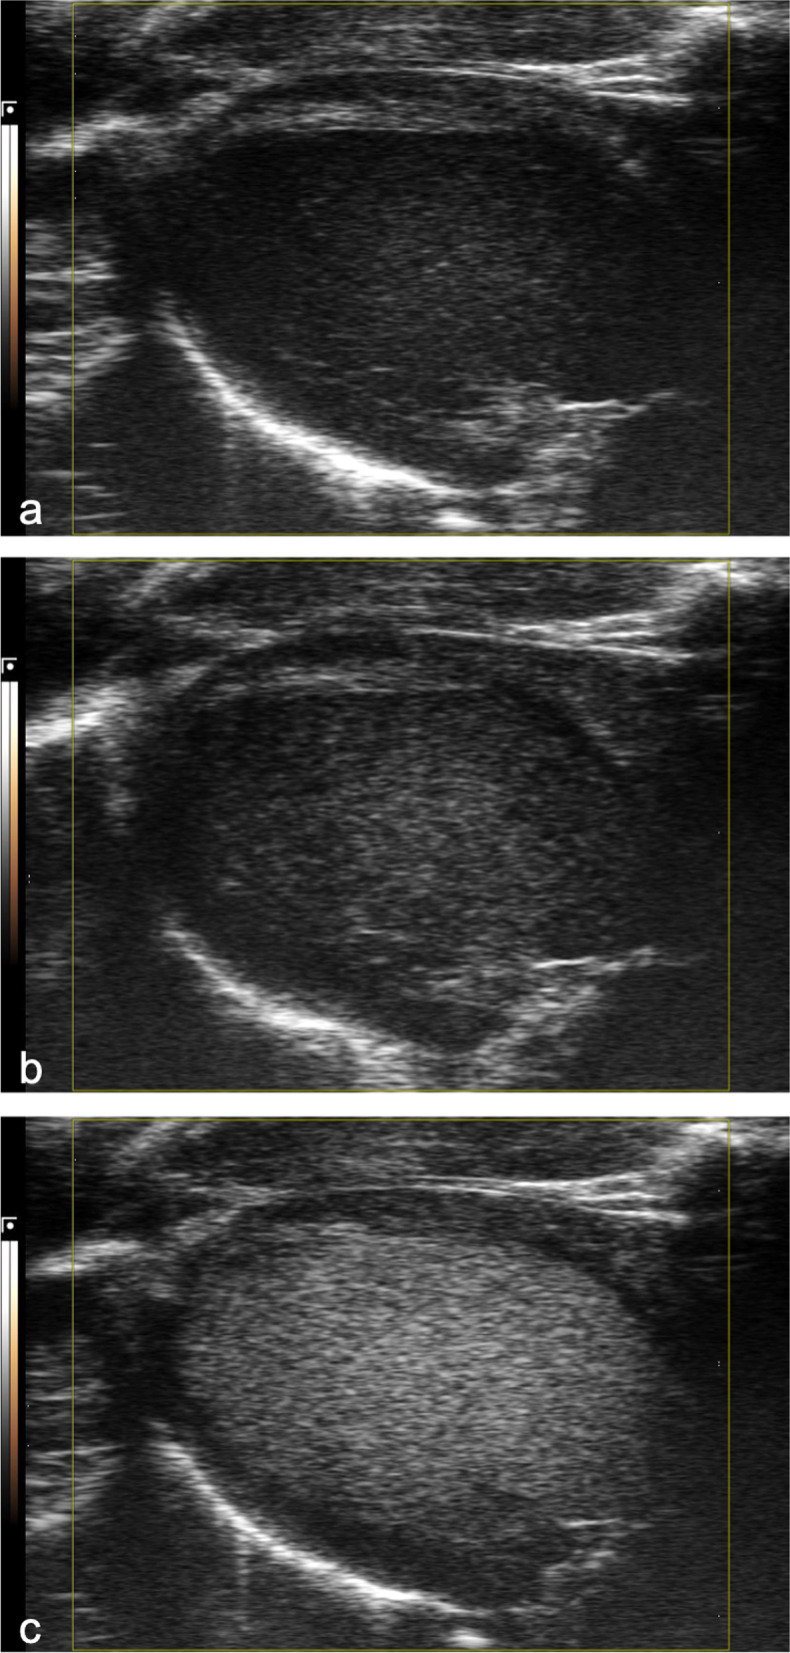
Representative time course of contrast activity in the lumen of the left ventricle. Shown are systolic gated modified parasternal long axis images that were acquired during nonlinear contrast imaging at the time of contrast agent arrival, *i.e.*, 0 s (a), 1 s (b), and 10 s (c) after contrast agent arrival
